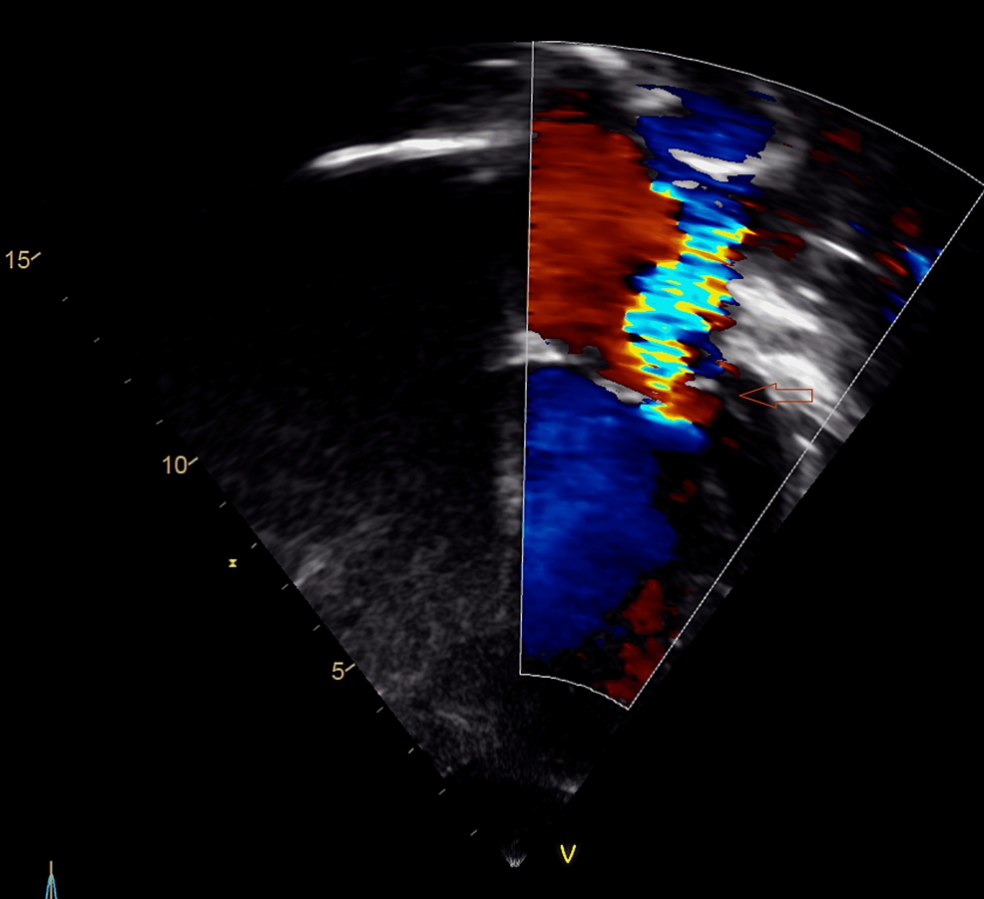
Echocardiography (Apical four-chamber view showing severe mitral regurgitation)
